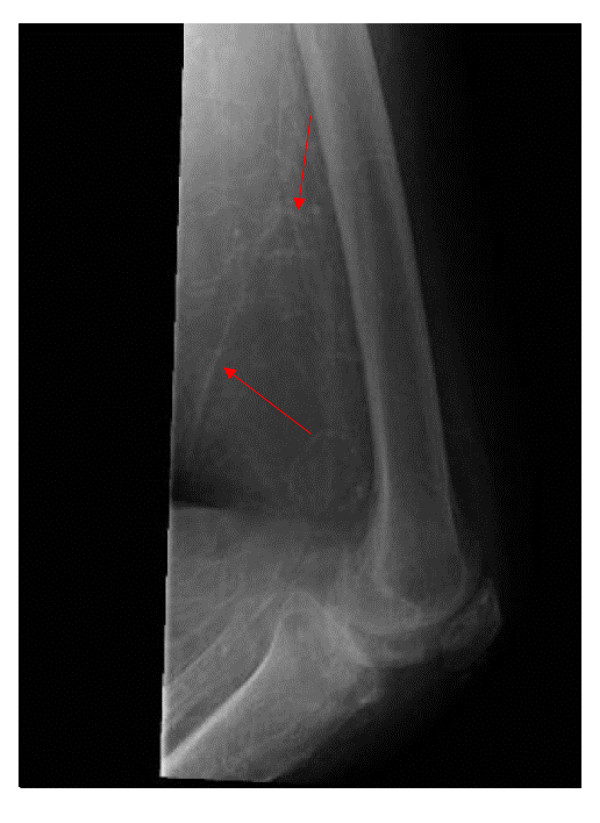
X-ray left thigh (lateral view) showing extensive vascular Calcification.
